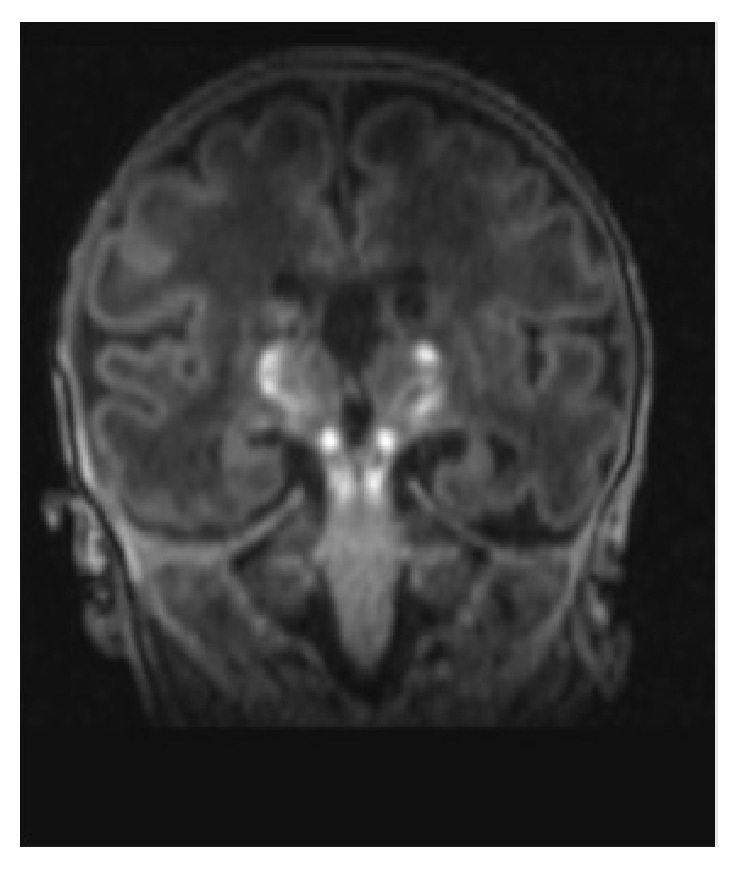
Postnatal cephalic MRI. T1 hyperintense pyramidal tract lesions.
